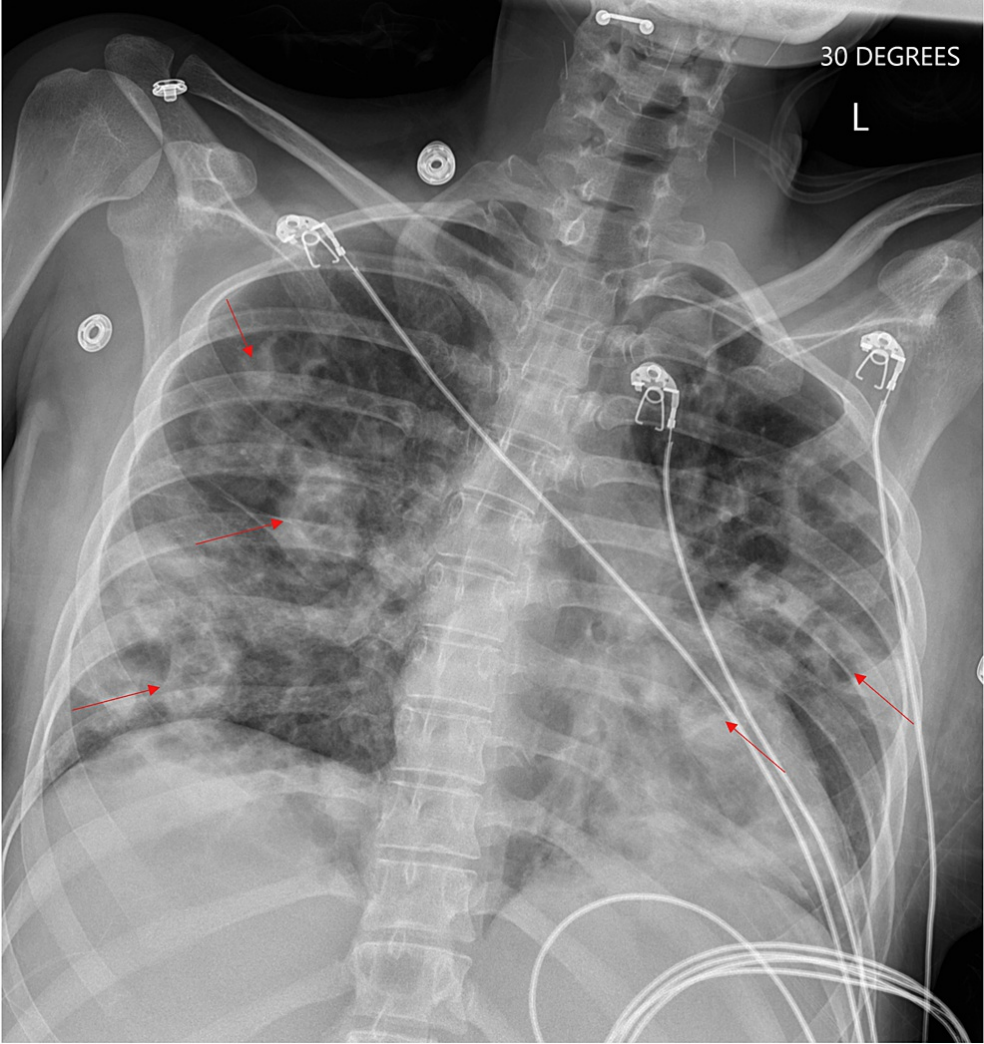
Portable 30 degrees upright anteroposterior chest radiograph demonstrates numerous bilateral lung opacities with central areas of lucency (red arrows). There is no obvious pleural effusion. The cardiac silhouette appears enlarged.
